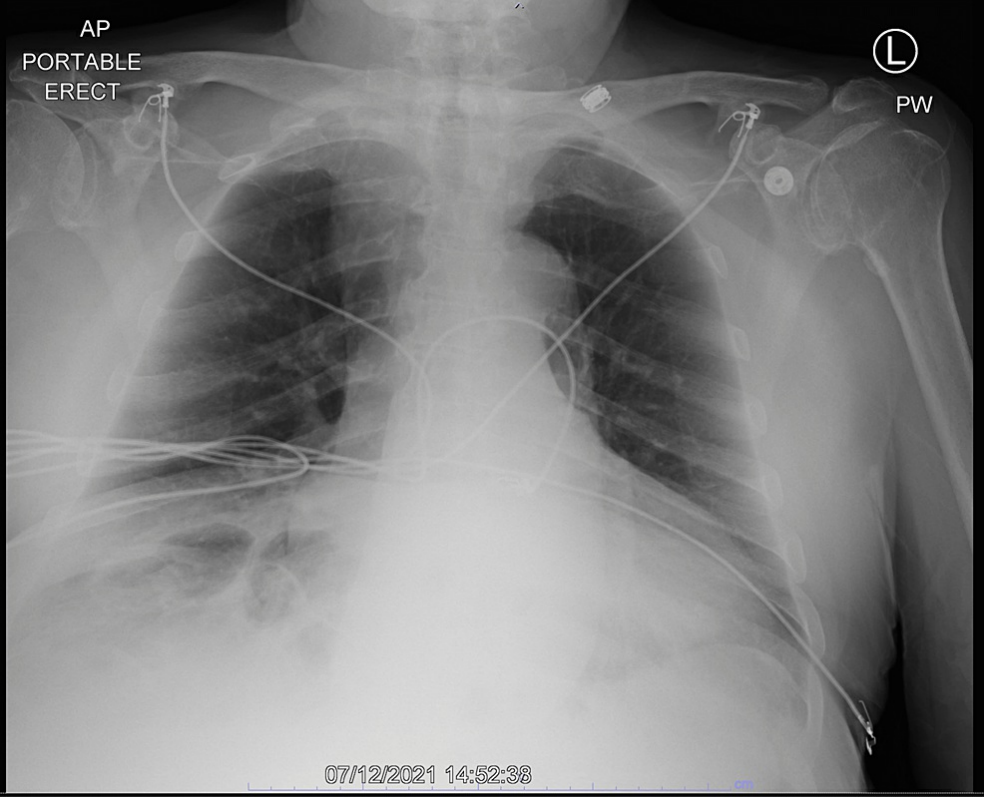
Initial chest X-ray. AP: Anteroposterior
